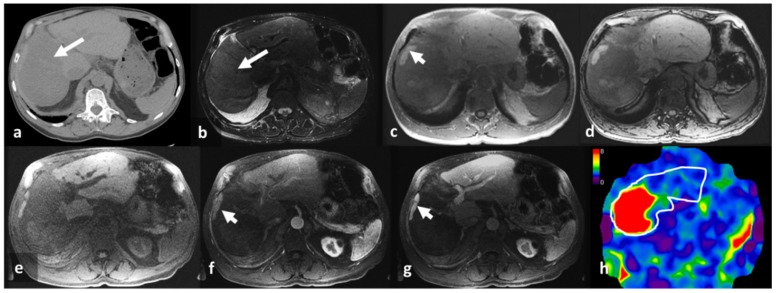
A 68-year-old male with known systemic amyloidosis and post autologous stem cell transplant on follow up. The axial non-contrast enhanced CT image (a) shows hypodense in the right lobe (arrow). The axial T2-weighted image (b) shows hyperintensity in the right lobe corresponding to the hypodensity in that lobe. In-phase (c) and opposed-phase (d) images show no significant signal loss (<5%) in the right lobe, confirming that there is no increase in fat. Note the small subcapsular area of sparing in the right lobe (arrowhead, (c)). The non-contrast enhanced (e), arterial phase (f), and portal venous phase (g) images show no significant enhancement in the right lobe but do show normal enhancement in the left lobe. The subcapsular focal area in the right lobe (arrow heads) shows similar enhancement to the left lobe, suggesting a spared region of normal liver parenchyma. The MRE stiffness map with a scale of 0–8 kPa (h) shows increased stiffness in the right lobe, corresponding to the abnormality seen on CT and magnetic resonance imaging (MRI), and normal stiffness in the left lobe. The mean ± SD LSM is 6.7 ± 1.2 kPa.
